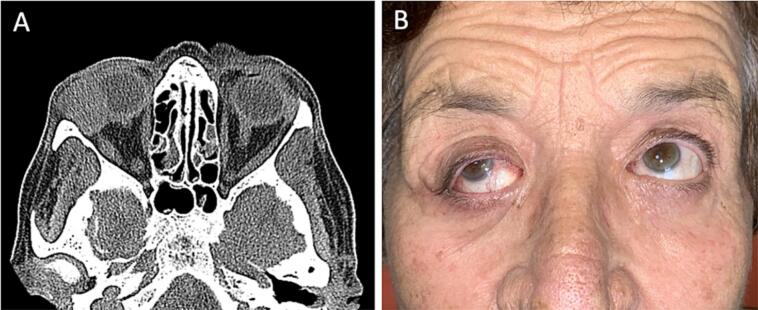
A) Computed Tomography: axial image showing a heterogeneous hyperintense mass, close to the lateral rectus muscle with inferior medial dislocation of the right eyeball. B) Preoperative view, showing ectropion with inferior medial dislocation of the right eyeball.
